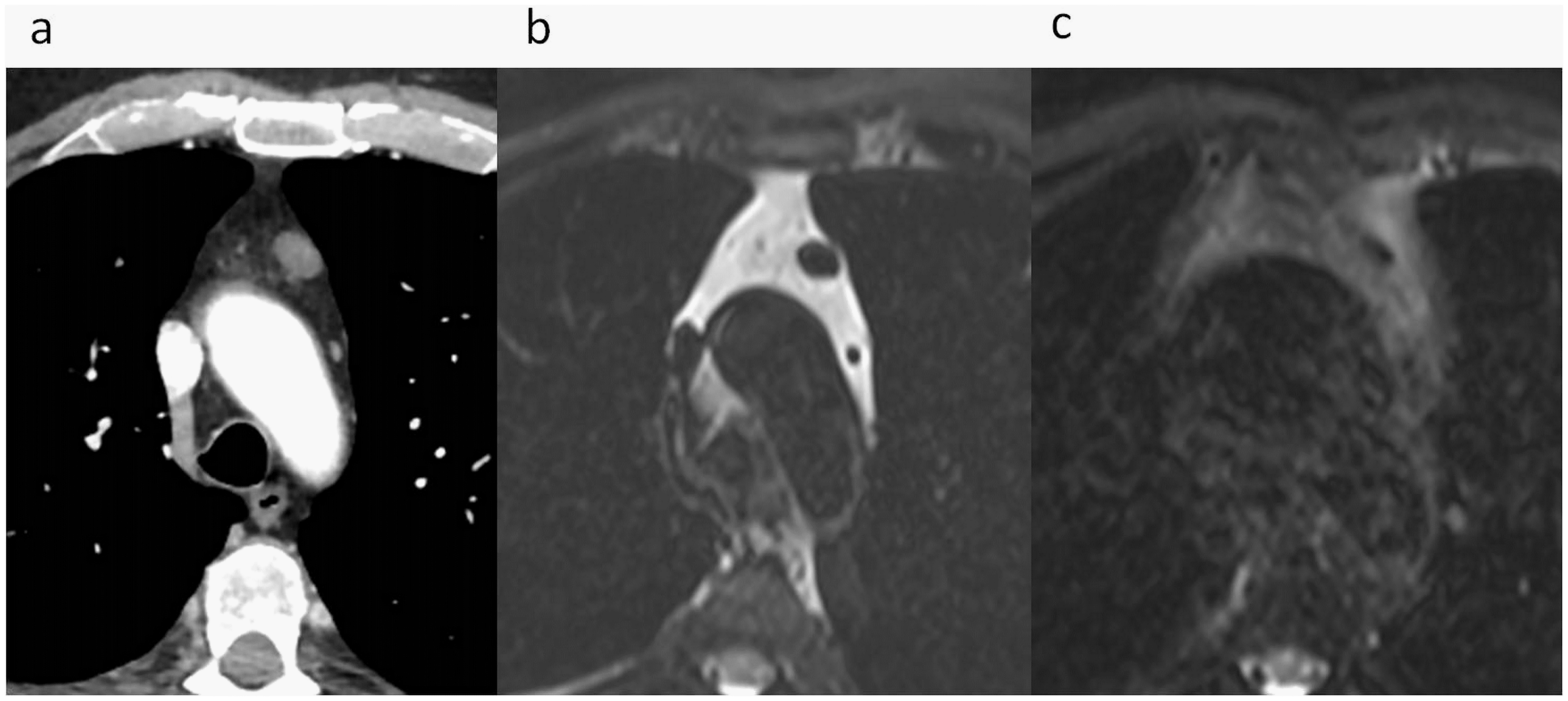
CT scan with iodine administration showing a nodule in the anterior mediastinum suspected being of thymic nature. (a). Fast spin echo T2 sequence at the same level with VP (b) and without (c). The thymic nodule is much better delineated under VP in b) than in c).
